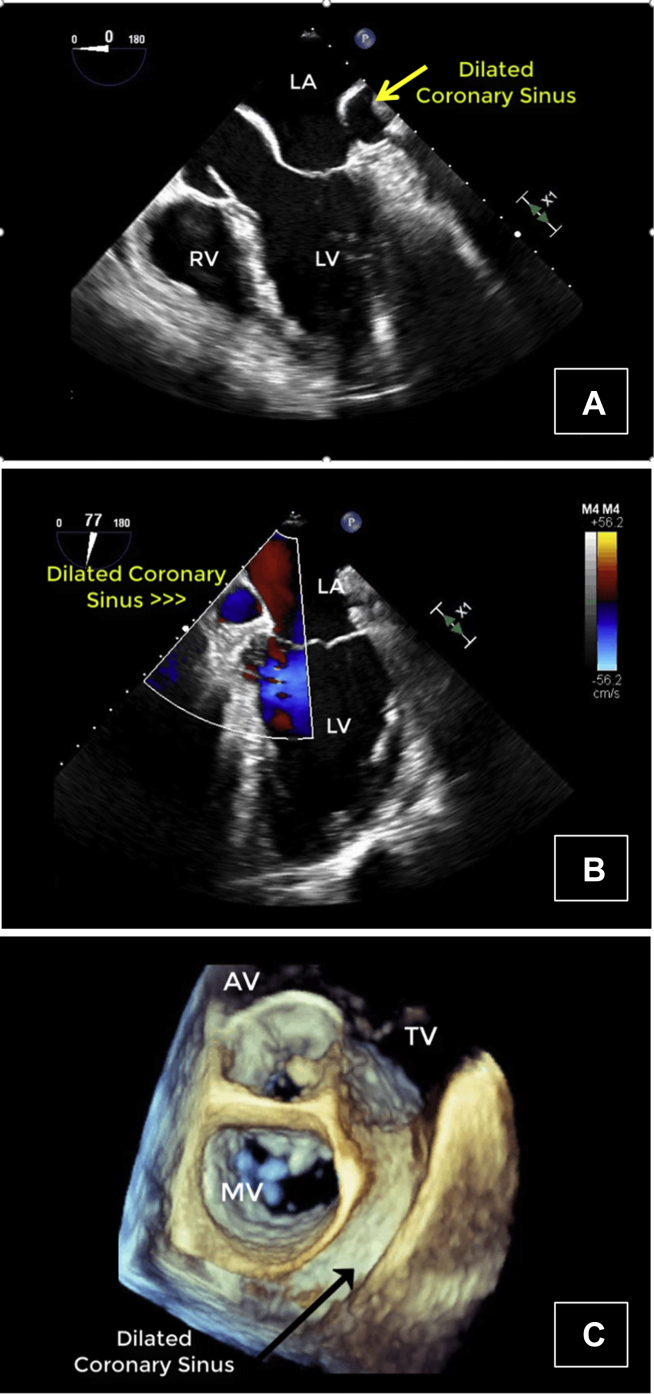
Case 5: R-SVC draining into the LA. Transesophageal echocardiogram with midesophageal view at 0° in grayscale (A), midesophageal view at 77° with color Doppler (B), and a three-dimensional zoom image in the so-called surgical view of the mitral valve (C). Transesophageal echocardiogram demonstrated a markedly dilated coronary sinus (A-C) with significant blood flow (B). As shown in other cases, a dilated coronary sinus suggests an atypical pattern of venous drainage and is usually consistent with diagnosis of a persistent L-SVC. Videos 6 through 8 correspond to this figure. *AV*, Aortic valve; *MV*, mitral valve; *RV*, right ventricle; *TV*, tricuspid valve.
